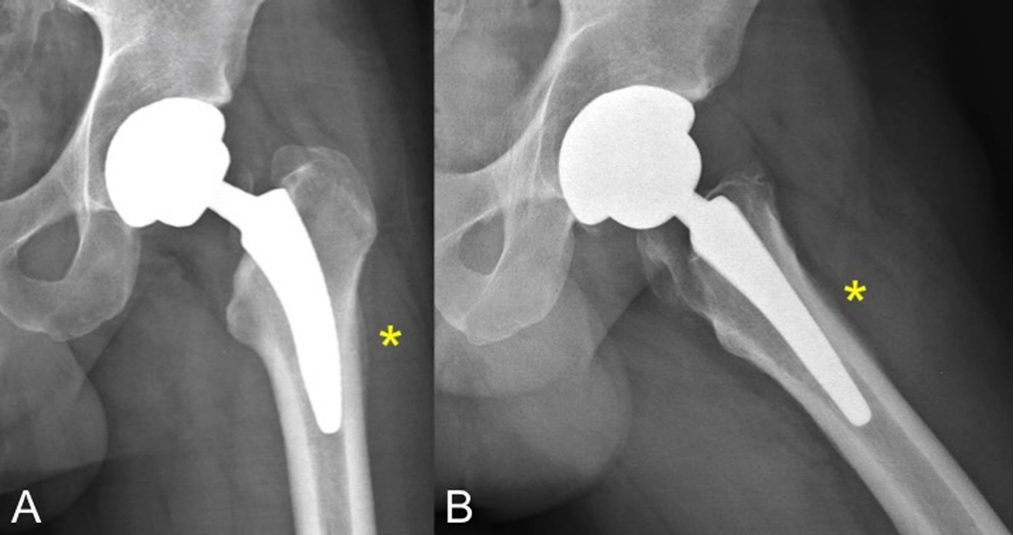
A and B. Anteroposterior (A) and lateral (B) x-ray views of the same patient's hip at 8 months of follow-up depicting bone remodelling and periosteal reaction located at the lateral and anterior femoral cortices (*), which correlated with unremitting pain that exacerbated with axial loading. No signs of prosthesis subsidence or loosening can be appreciated.
